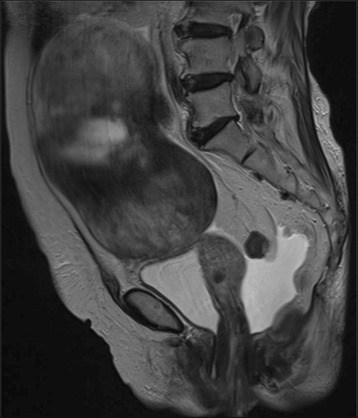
Magnetic resonance imaging of the pelvis before surgery for lung cancer. An abdominal mass with low signal intensity on T2-weighted images and ascites was observed
